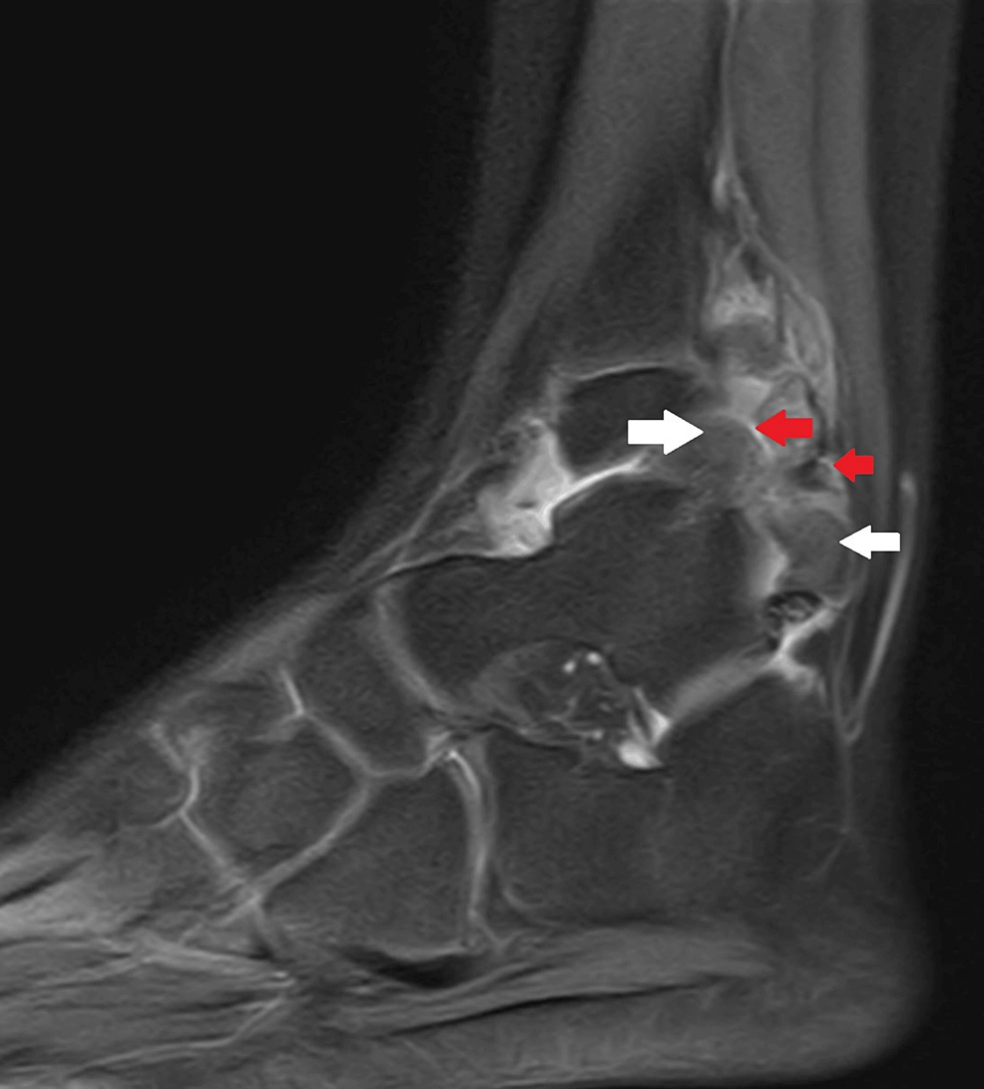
MRI proton density fat-suppressed sagittal images demonstrate the osteochondromas (white arrows) in the interosseous region. The thin cartilaginous cap of the osteochondromas can be seen as a hyperintense signal (red arrows)
